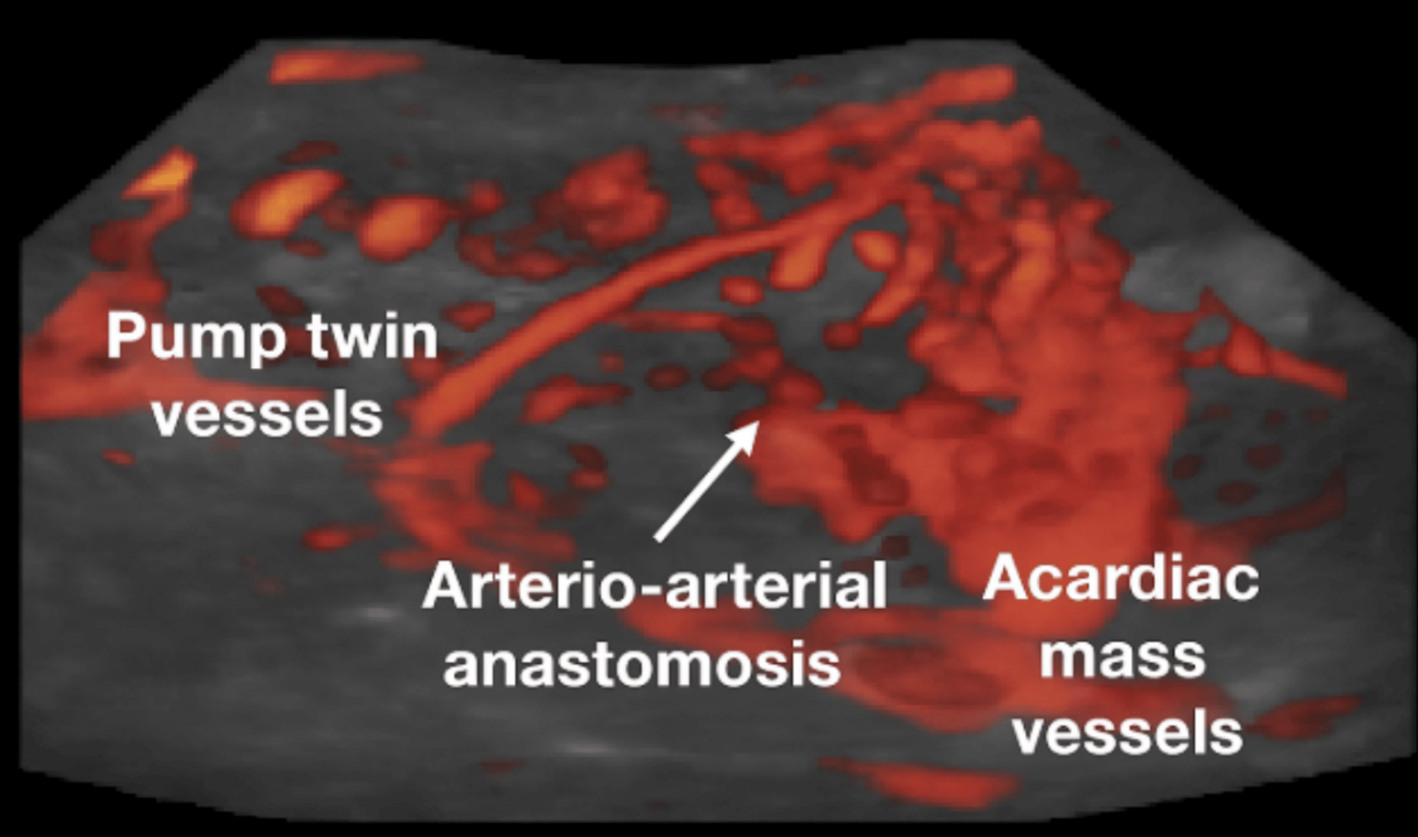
Three-dimensional (3D) power Doppler angiography with glass-body rendering.
